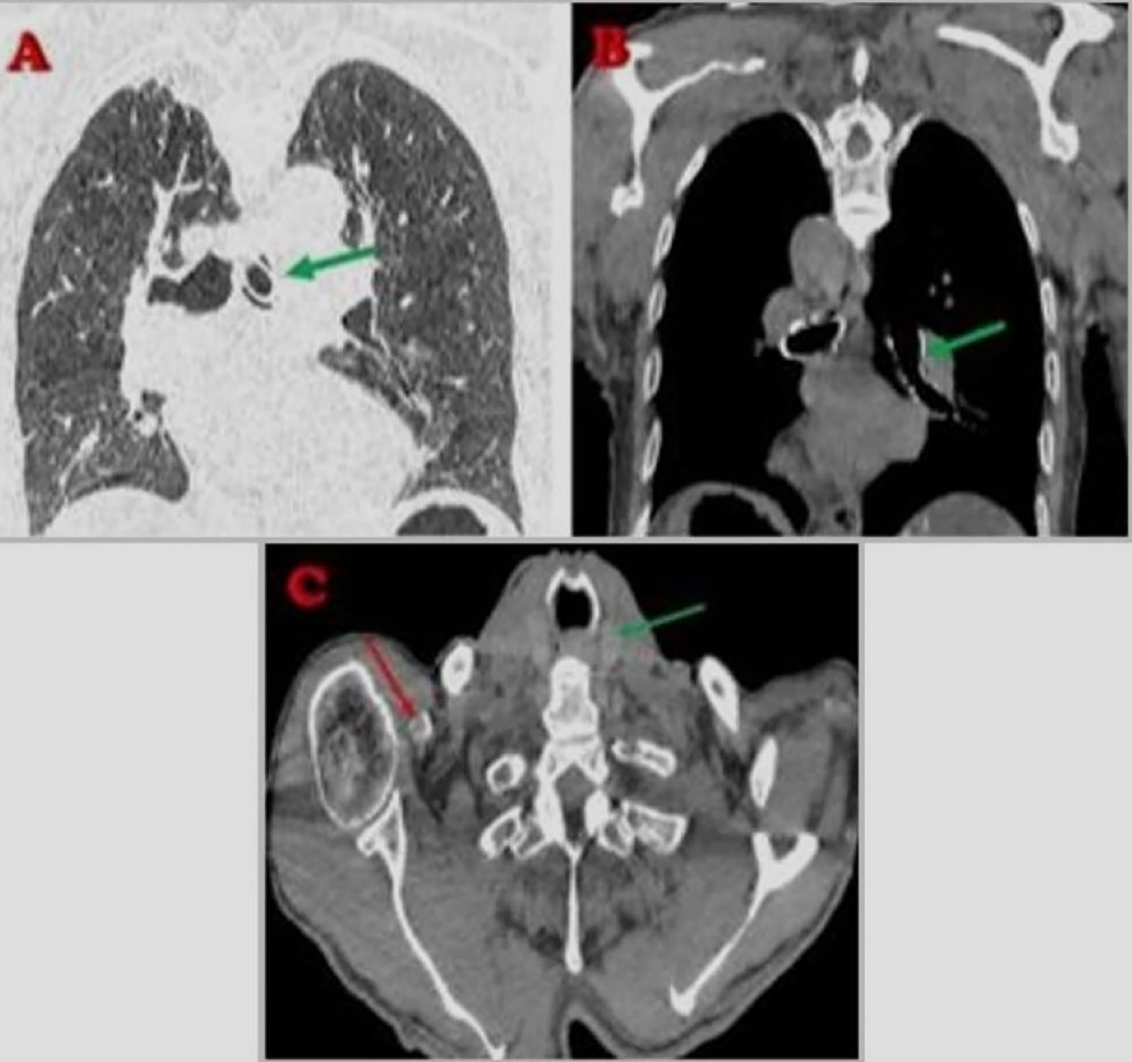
CT-Scan showing tracheostomy tube misplacement (green arrow), mediastinal shift, and pacemaker position (red arrow).
